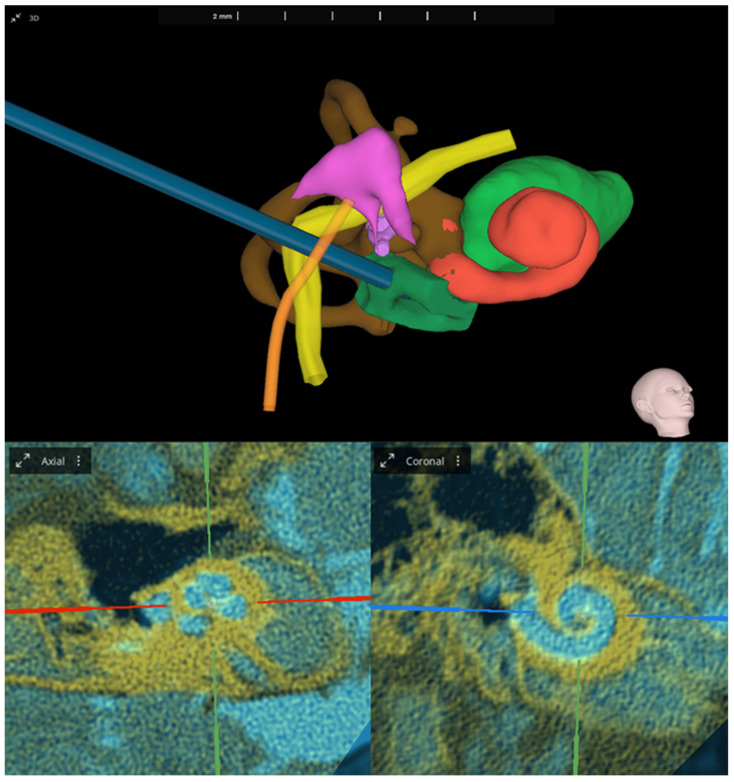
(Top): Three-dimensional inner ear reconstruction with optimal trajectory calculation. Shown anatomical structures: cochlea (red), inner auditory meatus (light green), vestibulum and semicircular canals (brown), facial nerve (yellow), chorda tympani (orange), ossicle chain (purple), and bony overhang (dark green). (Bottom): Image fusion showing 0.60 mm CT (yellow) and T2 weighted MRI (blue) in the oblique coronal view. Analyses and images were generated with OTOPLAN V4 (3.0.0).
